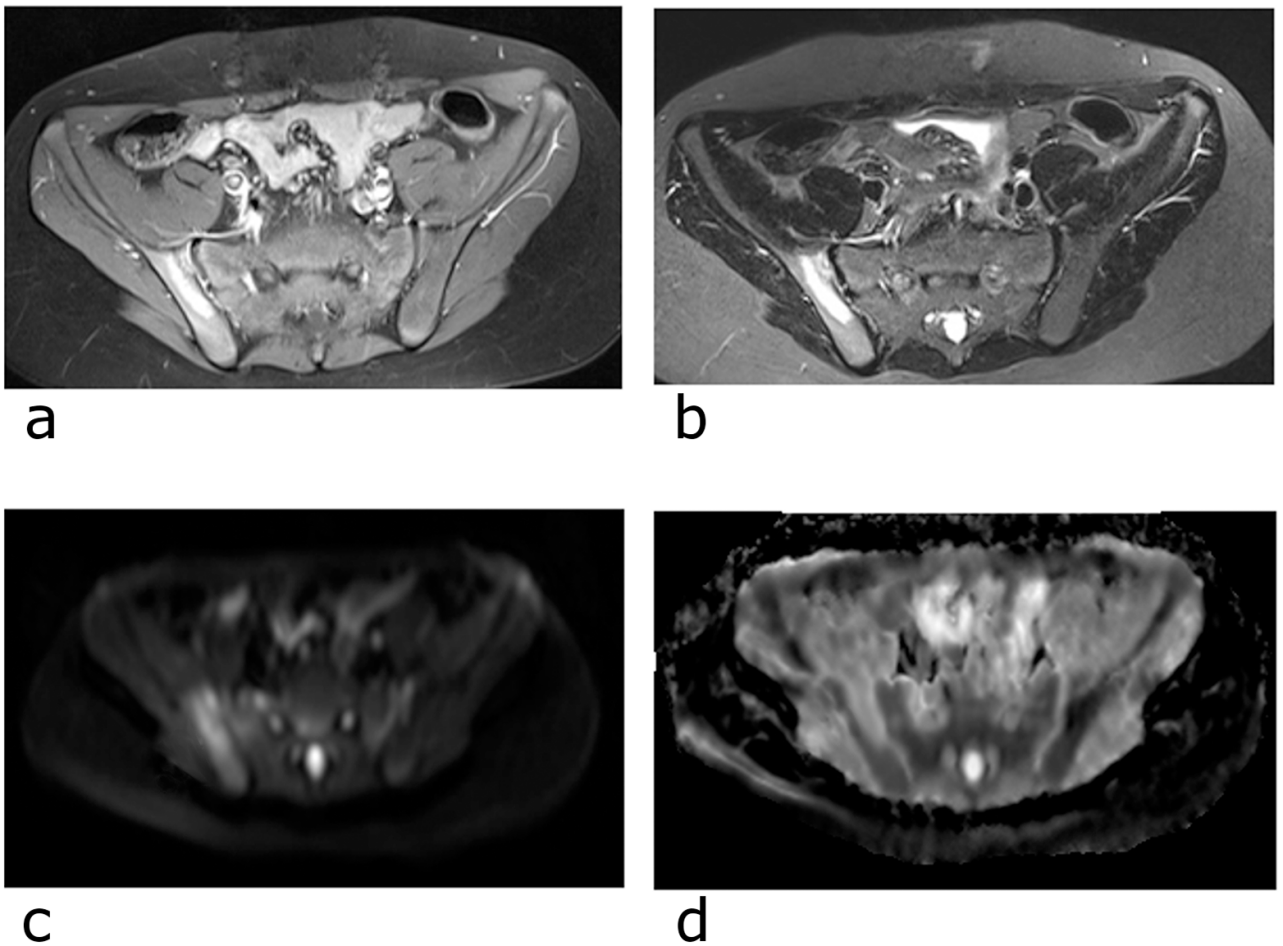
Multiparametric MRI. a) contrast-enhancement of a CRMO-lesion in the right Os ilium on axial T1-TSE FS b) corresponding signal alteration on STIR images c) correlating signal changes on axial DWI b800 d) and high signal intensity on ADC.
